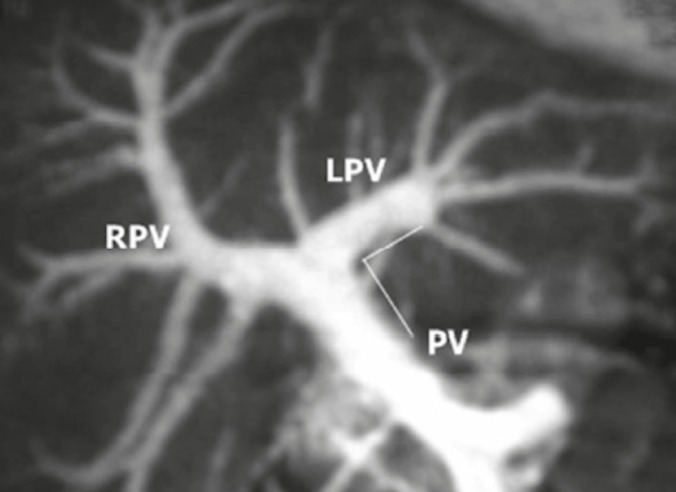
CT portography of Case 2 showing adequate length of the right portal vein and satisfactory angle between the main and left portal veins
